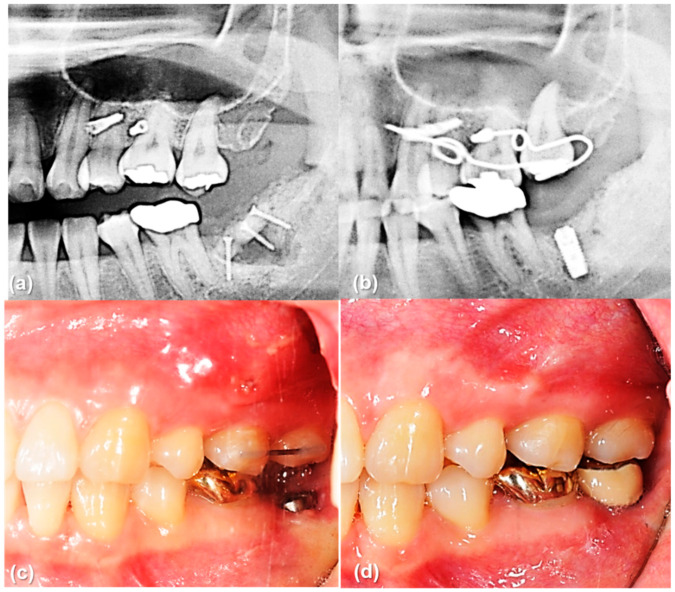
The orthodontic miniscrew supported the counterclockwise rotational force in a patient with extruded upper left second molar: (a) Pretreatment panoramic radiograph shows extrusion of upper left second molar. (b) Posttreatment panoramic radiograph shows sufficient intrusion of upper left second molar after six months of treatment. Dental implant was placed in the lower left second molar area. (c,d) Intraoral photographs before and after implant crown treatment.
